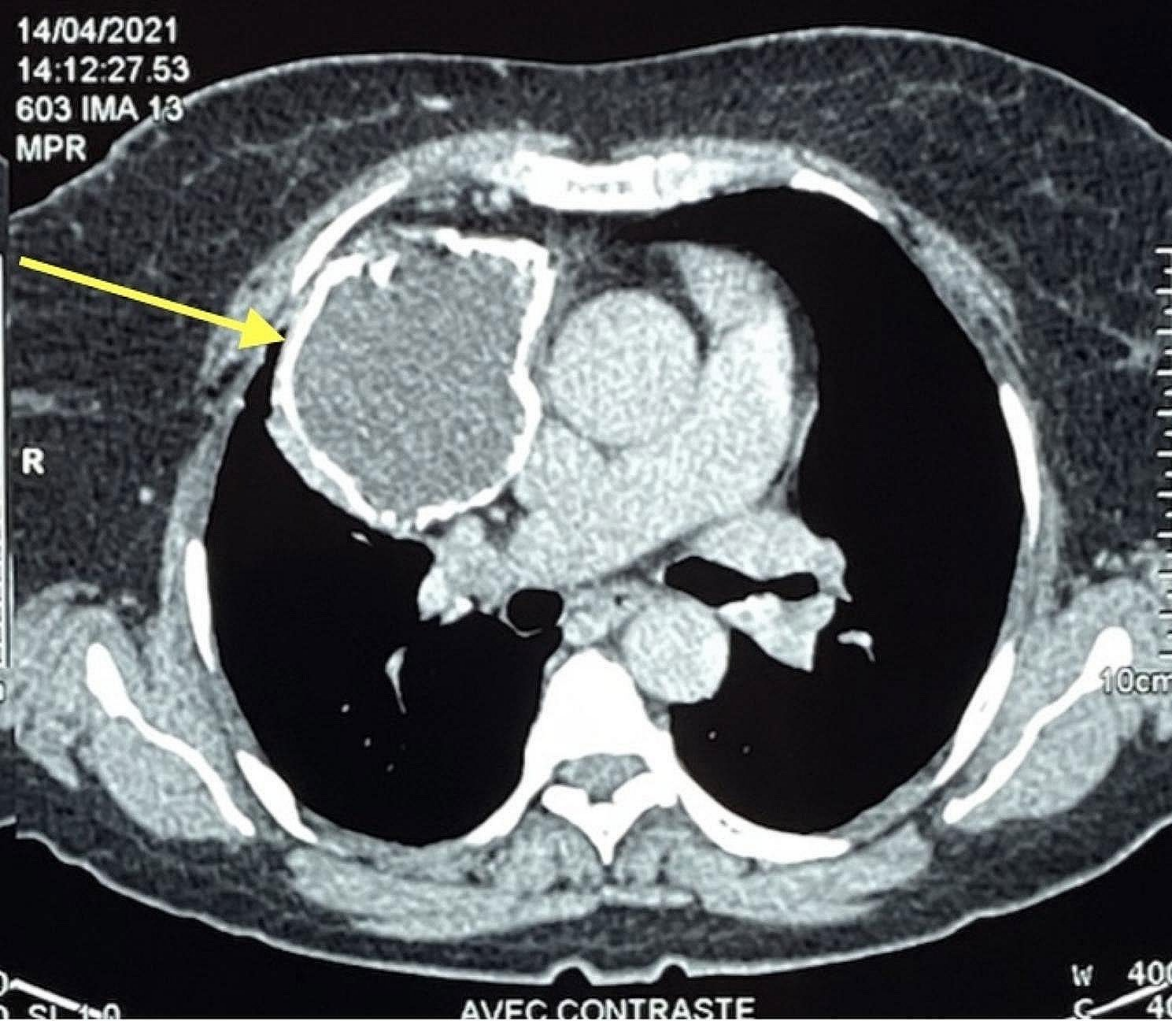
CT scan image of the right mediastinal mass (Yellow arrow)
